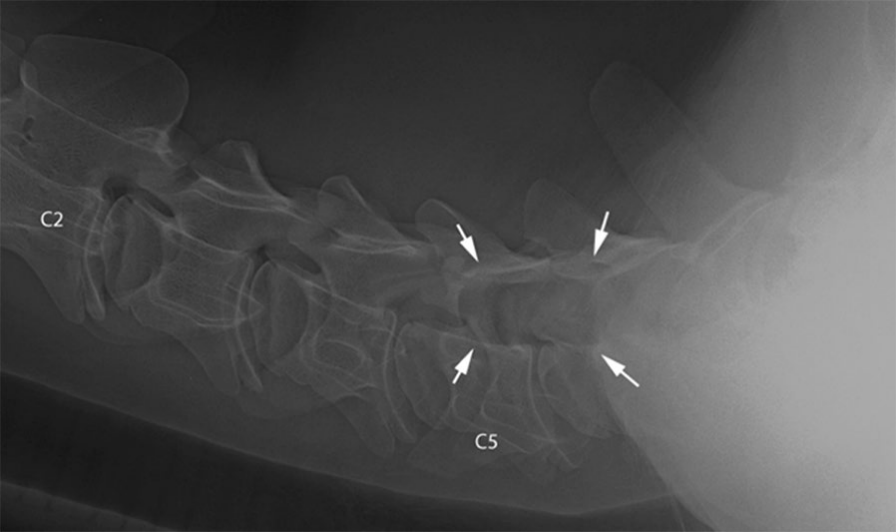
Laterolateral radiographic view of the cervical vertebral column. Laterolateral radiographic view of the cervical vertebral column of a 9.5-month-old Red Holstein heifer. The second (*C2*) and fifth (*C5*) vertebrae are labelled. A lytic lesion at the level of C5 and C6 (*arrows*) superimposed on the vertebral canal corresponds to the lateral parts of these vertebrae. The lesion has a thin sclerotic rim cranially
